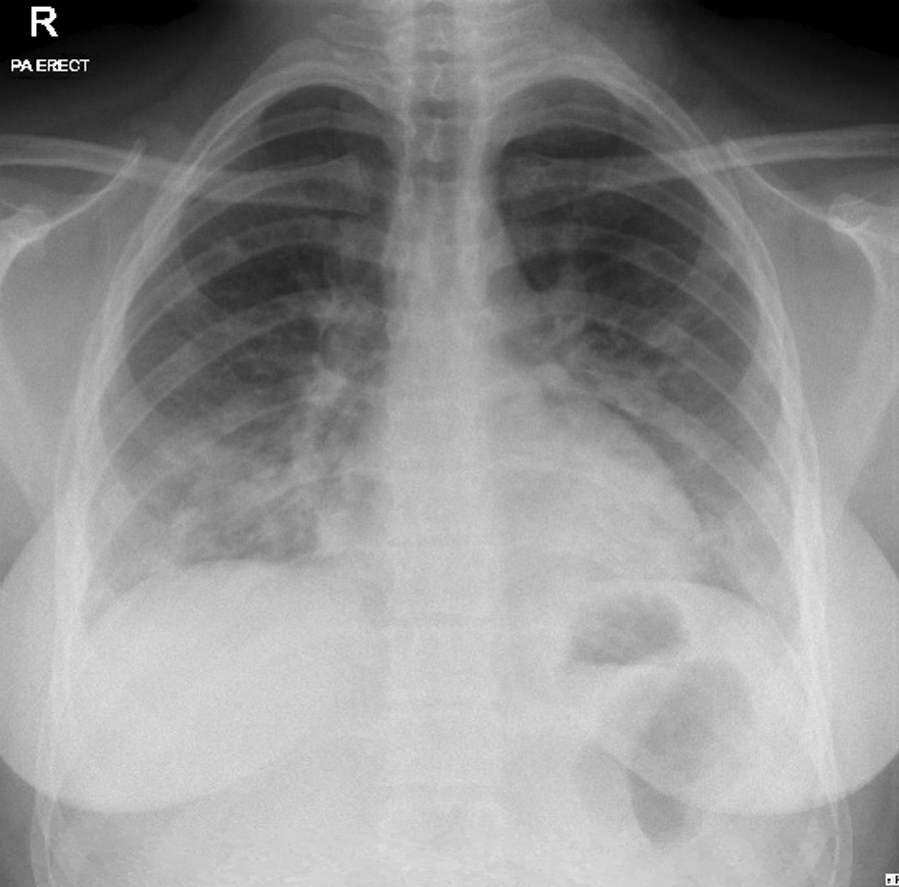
A 16-year-old girl with COVID-19 pneumonia based on positive RT-PCR test who presented with fever and cough for 3 days. Frontal chest radiograph shows bilateral multifocal ground-glass opacities and consolidations. The patient’s serum biochemical parameters show a significantly elevated LDH level of 260 U/L
